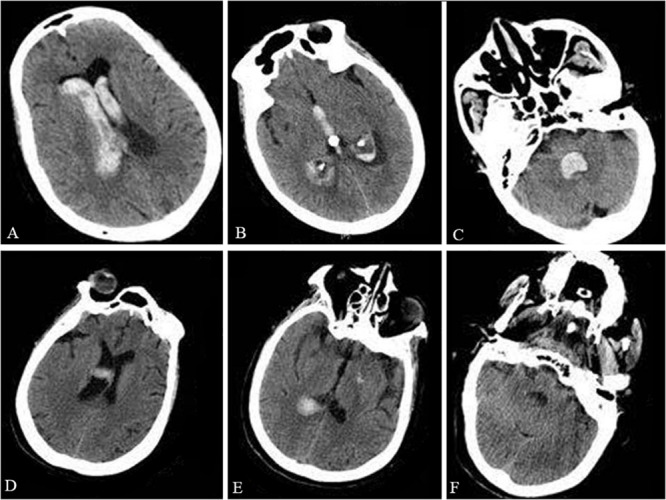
Preoperative and postoperative CT. The preoperative head CT showed intraventricular hemorrhage in the lateral ventricles (A), third ventricle (B), and fourth ventricle (C). The postoperative head CT taken during the patient’s acute respiratory failure and shortness of breath excluded an intracranial re-hemorrhage in the lateral ventricles (D), third ventricle (E), and fourth ventricle (F).
